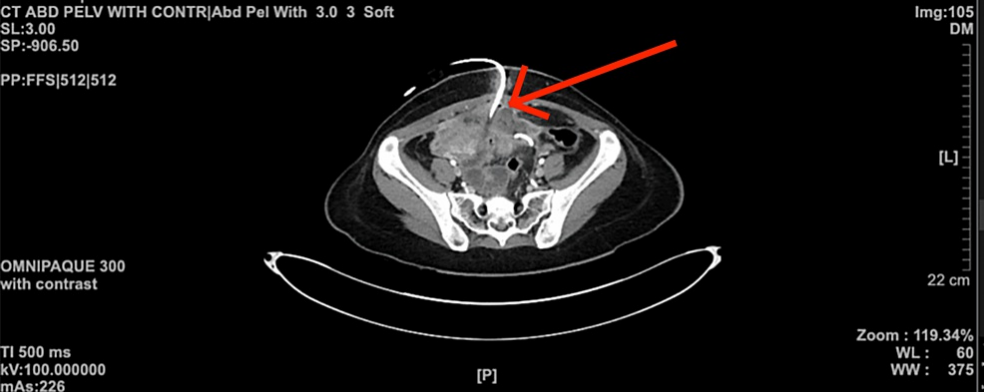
CT scan of the abdomen and pelvis with contrast conveying a pigtail (red arrow) inserted in the region where the abscess is found CT: computed tomography
